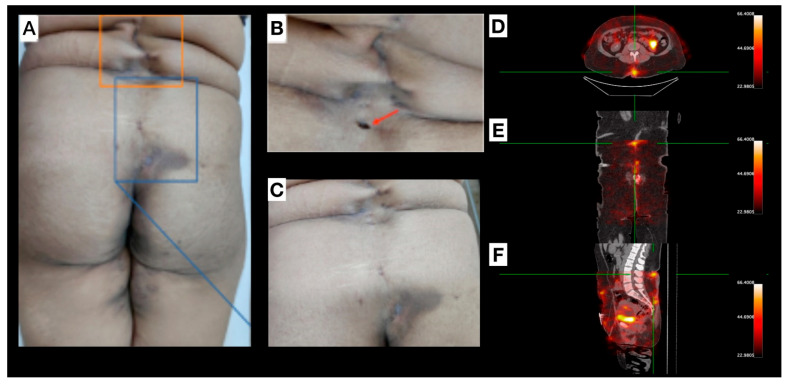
Patient 4 (P4): (A–C) depict lesions located within the lumbar and gluteal regions, originating from the L1 vertebral level and extending caudally to the sacral area. Interspersed within these affected areas, regions of apparently healthy tissue are observed, separating the discernible scars and clinically quiescent lesions. Image (B) highlights explicitly an oval-shaped opening (red arrow) representing a previous fistulous tract. A pilonidal cyst is delineated in the sacral region, with optimal visualization in image (C). (D–F): [^99m^Tc]Tc-anti-TNF-alpha SPECT/CT shows, in axial (D), coronal (E), and sagittal (F) views, abnormal uptake foci in the lumbar region, pilonidal cyst, and in a clinically inactive lesion (green reticle).
